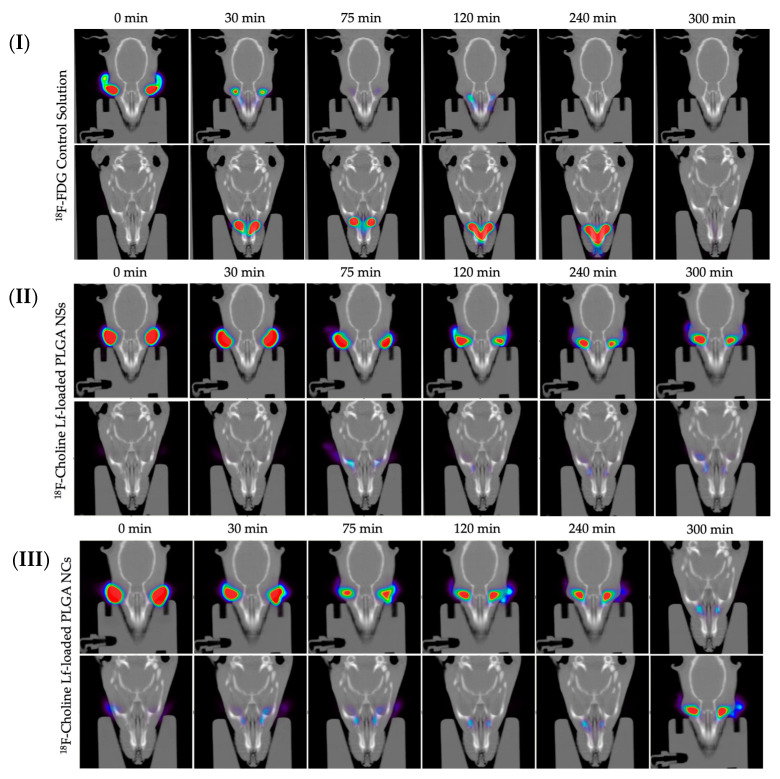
Fused PET/CT images during the 5 h studied interval. (I) ^18^F-FDG buffered aqueous solution, (II) ^18^F-Choline radiolabeled PLGA NSs and (III) ^18^F-Choline radiolabeled PLGA NCs.
